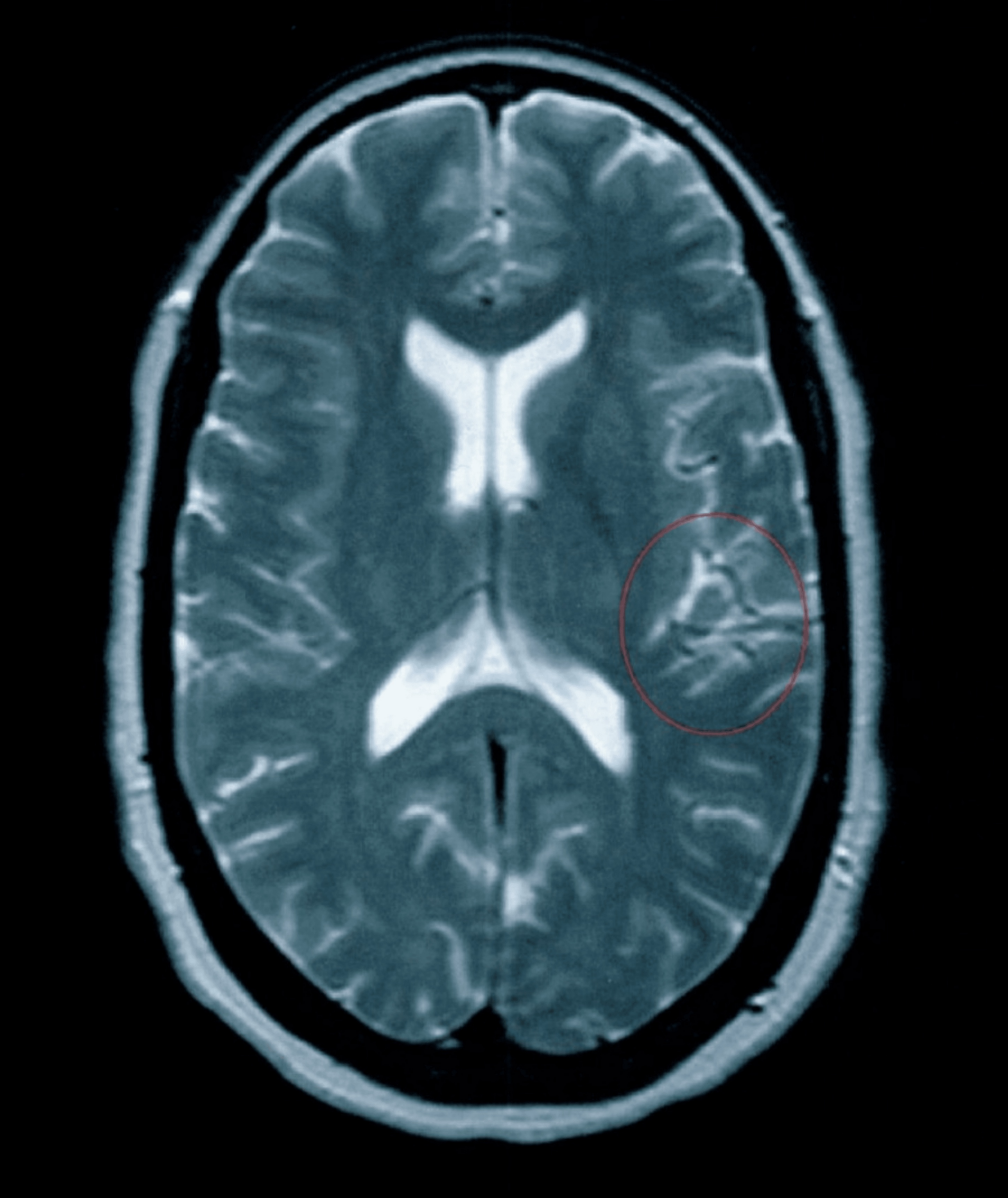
Post-resection MRI of the brain from April 2018 The surgical area is marked with a circle.
